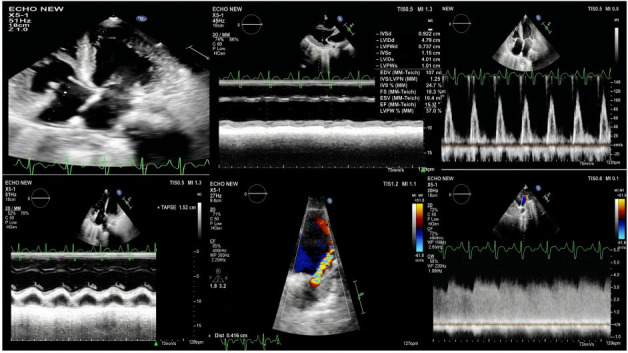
2D Echocardiogram of a child with atransferrinemia showing severe biventricular systolic dysfunction, LV diastolic dysfunction, and an incidental 4 mm-sized PDA.
